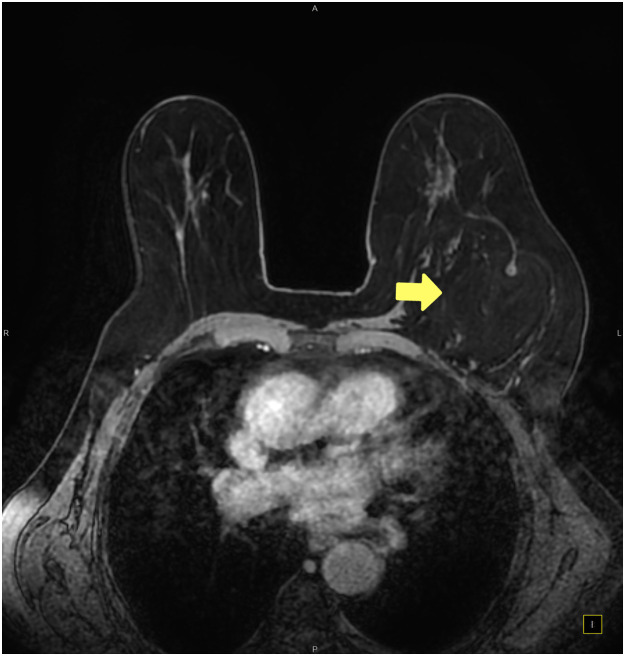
Preoperative MRI. The yellow arrow shows a formation between major and minor pectoral muscles measuring approximately 60 × 80 × 130 mm in the left breast. Notably, contrast-enhancing foci, reminiscent of parenchymal tissue, are observable bilaterally across the breasts.
